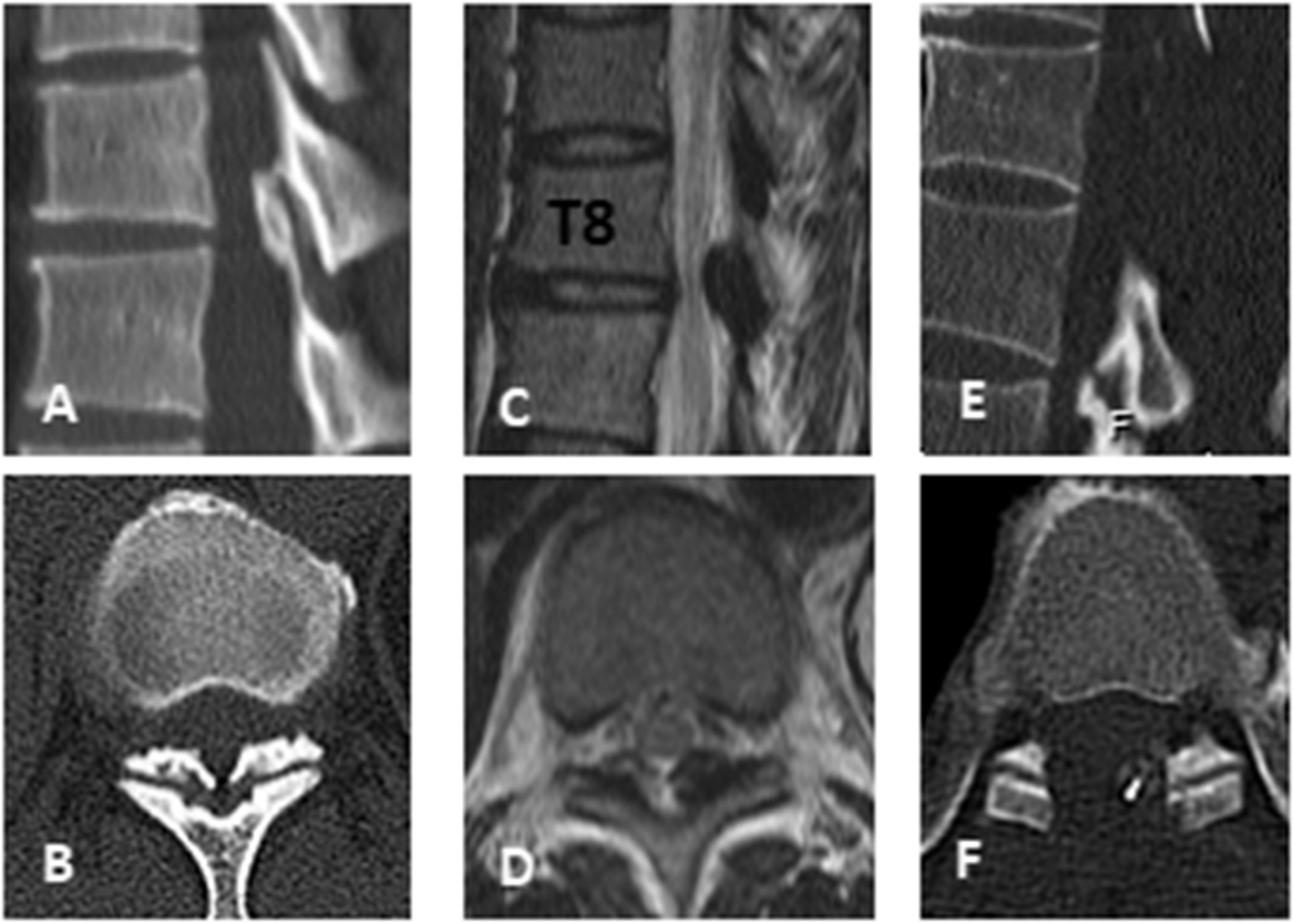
Laminectomy alone. A 55-year-old female patient, ossification of ligamentum flavum and compression degeneration of spinal cord could be seen on CT (figure A, B) and MRI (figure C, D) before operation. We performed OLF resection with LA (Fig. E, F). During the 2-year follow-up, the preoperative JOA score increased from 7 to 8.5 (JOA score improvement rate was 37.5 %)
